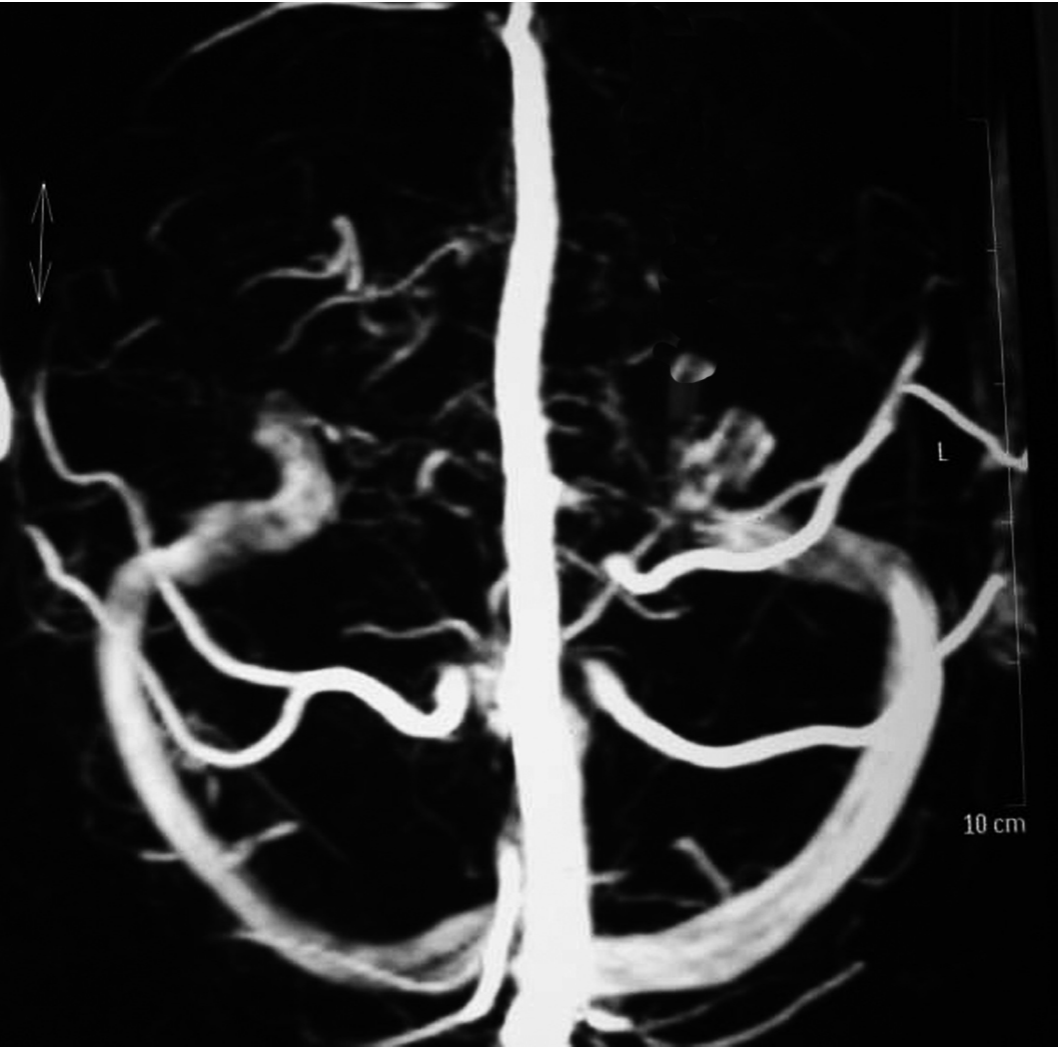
Brain MRI Venous Angiography. Normal.
